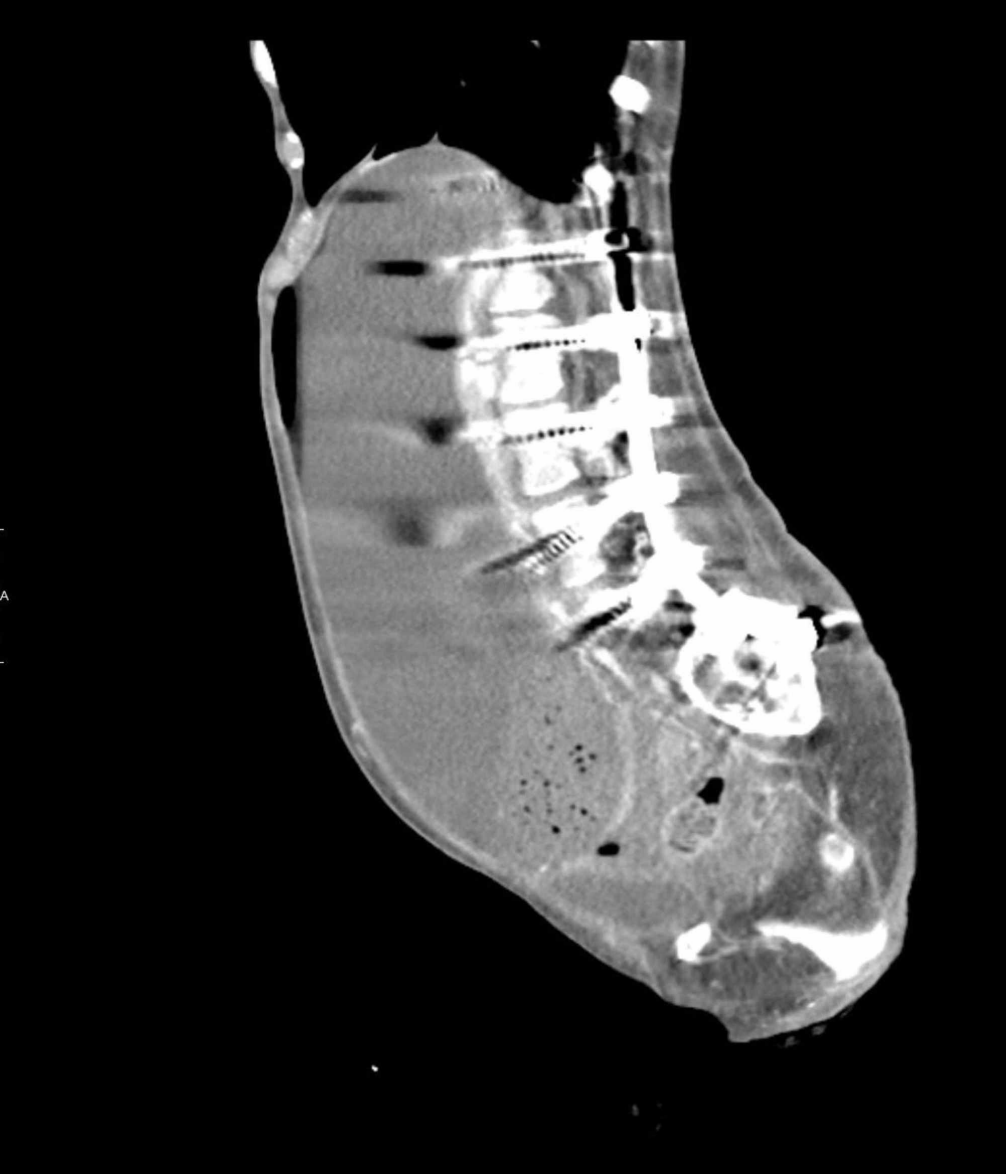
Computed tomography (CT) scan of the abdomen (sagittal section) with contrast showing massively distended stomach extending into the pelvis
